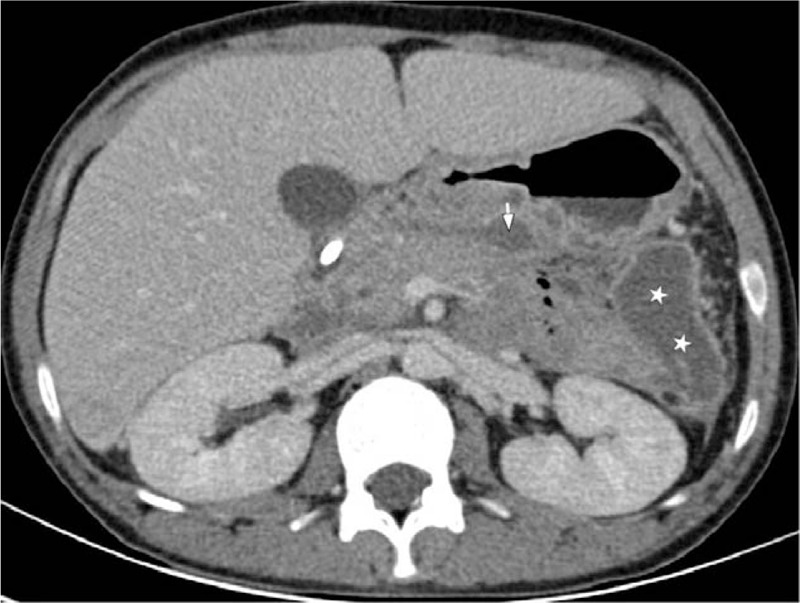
Abdominal contrast-enhanced CT displaying decreased peripancreatic necrotic fluid collection compared with Figure 2B (white arrow) and defined inflammatory wall (white stars).
